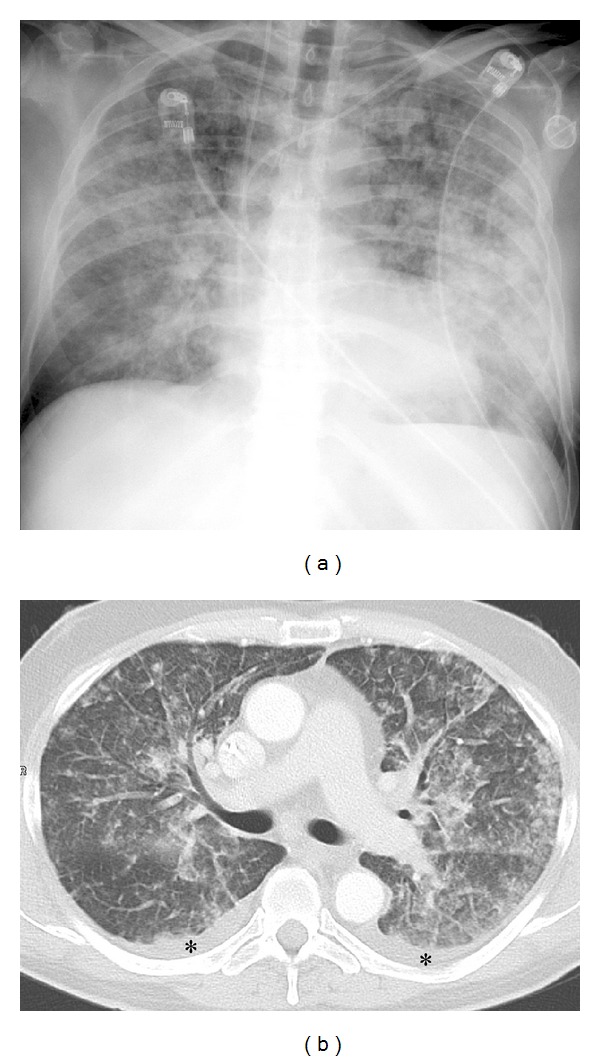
X-ray (seven days after inpatient admittance) and CT scan of the chest (five days after initiation of chemotherapeutic treatment). Patchy airspace consolidation with peribronchial and peripheral distribution. Small pleural effusion bilaterally (black asterisk).
